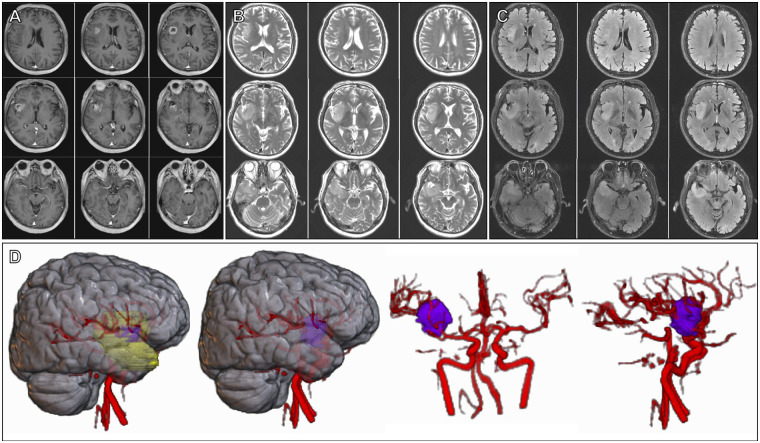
Multimodal image acquisition and 3D model reconstruction. Preoperative MRI indicated a space-occupying lesion in the right insular lobe with significant enhancement [(A), T1CE], and obvious edema areas could be seen around it [(B), T2WI and (C), FLAIR]. Through multimodal image fusion and 3D model reconstruction, the spatial positions of the enhanced part of the lesion (blue) and the non-enhanced part of the lesion (yellow) can be visually located, and the lesion is closely related to the lentiform artery perforation branch of the middle cerebral artery [(D), 3D model].
